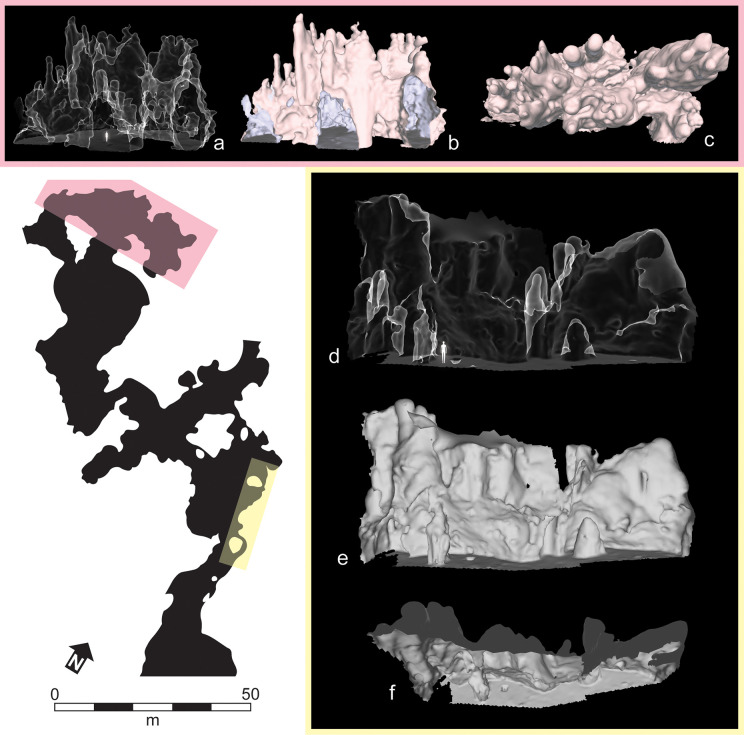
3D models of selected wall sections within PYS. Northern (pink, a–c) and central (yellow, d–f) eastern walls. Each section was represented using x-ray style (a, d) and radiance scaling rendered shaders (b–c, e–f). The northern section shows a cave chamber with three separate openings and a roof consisting of a series of cupolas. The central east section displays similar cupolas with sporadic stalagmites and stalactites.
